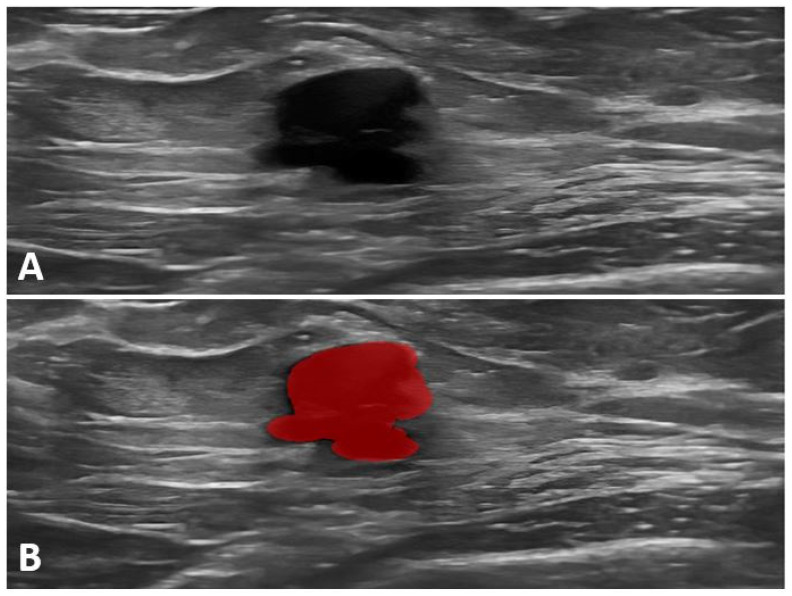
BRCA1*-positive patient. (A) Ultrasound image showing a hypoechoic, irregularly shaped mass with a partially microlobulated and indistinct margin. (B) Ultrasound image with segmented tumor area highlighted in red.
